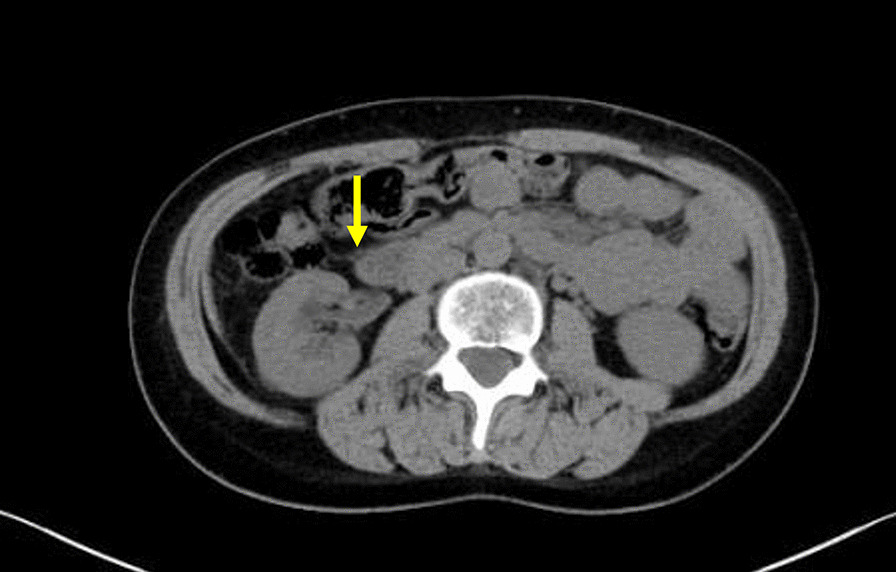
Axial computed tomography scan image of the right kidney 2 years after radiotherapy. Hydronephrosis improved (yellow arrow)
